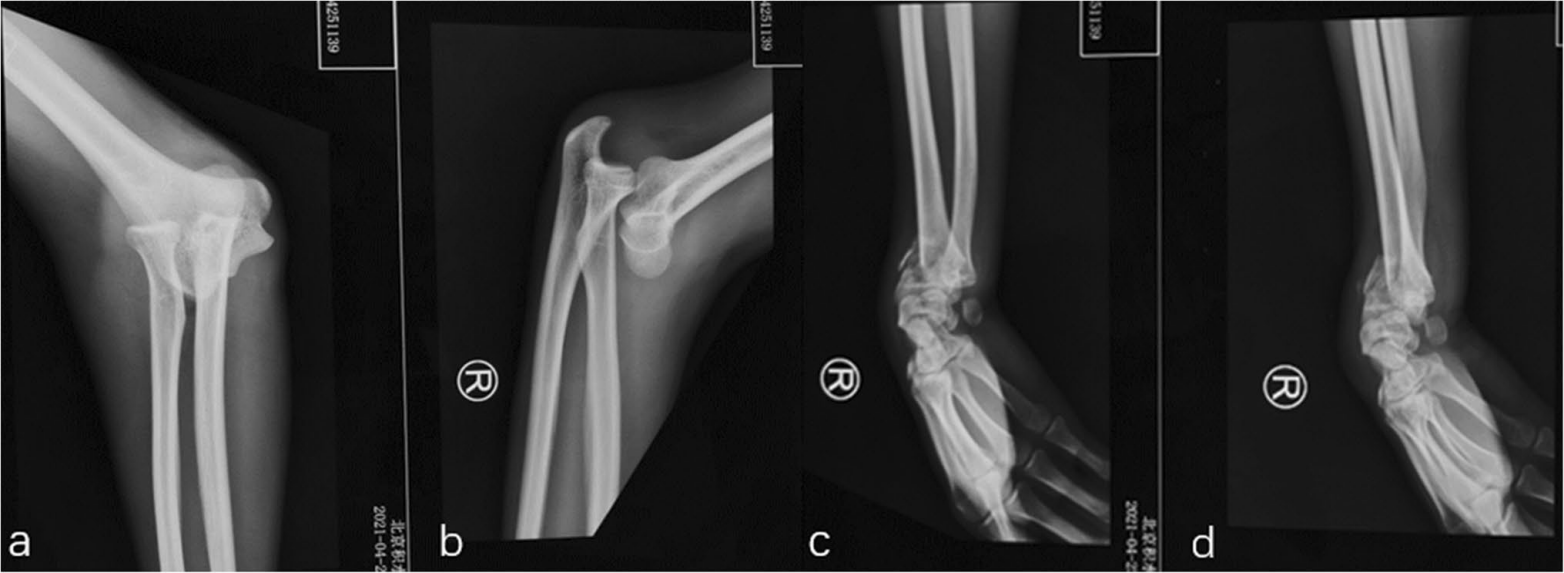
Pre-operative radiographs show a divergent dislocation of the elbow (a–b) combined with a distal radius fracture (c–d)
